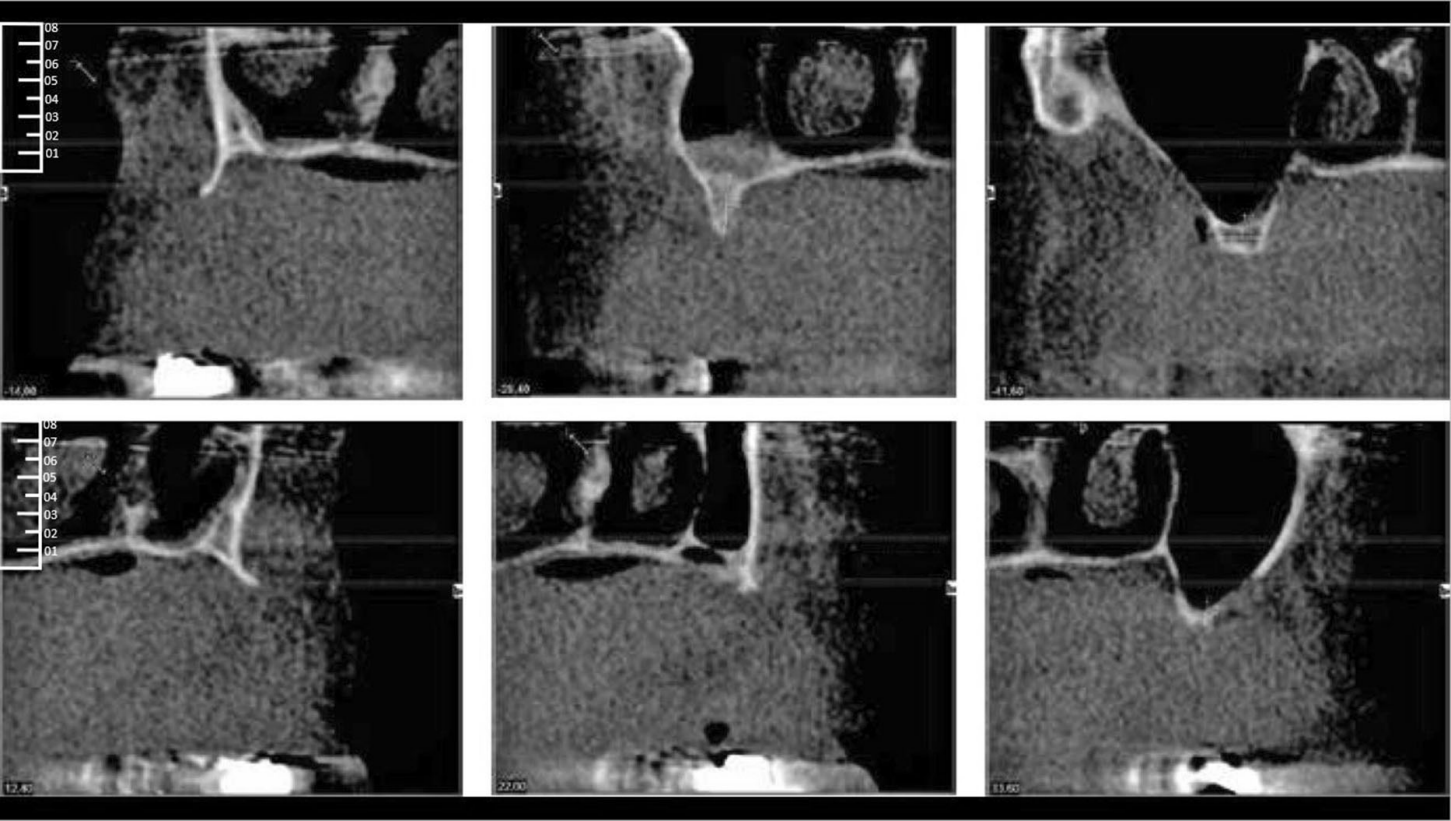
Transversal CBCT-Slices of the maxillary bone dimension before augmentation (region: 012, 015, 017 upper rows, 022, 024, 026 lower row)
